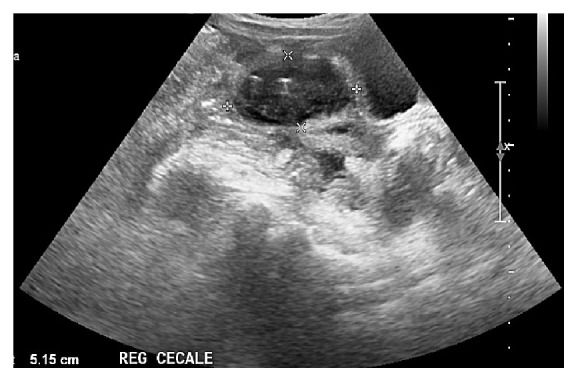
Residual pelvic abscess ultrasound image in OA group.
